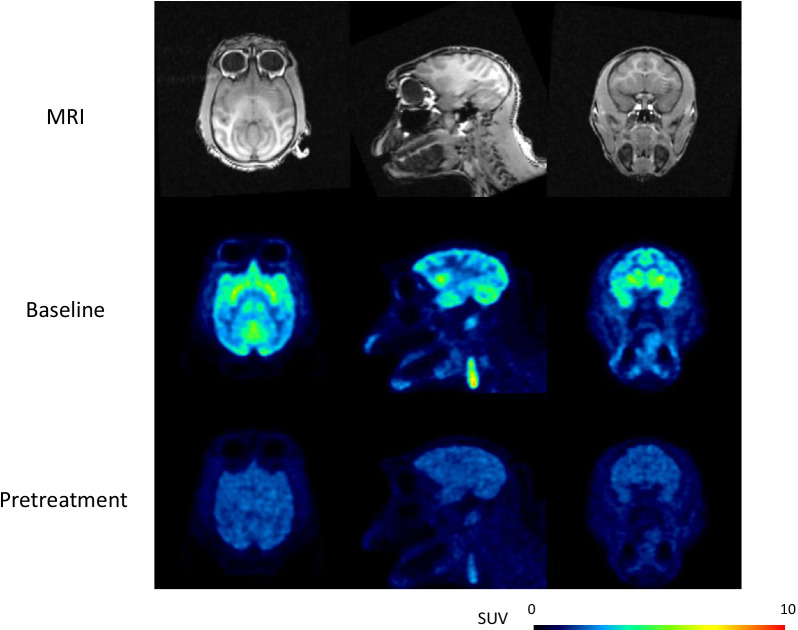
Representative MRI and PET summation images (0–63 min) of [^11^C]PF-06809247 at baseline and following pretreatment with a selective MAGL inhibitor (NHP4; 0.42 mg/kg)
